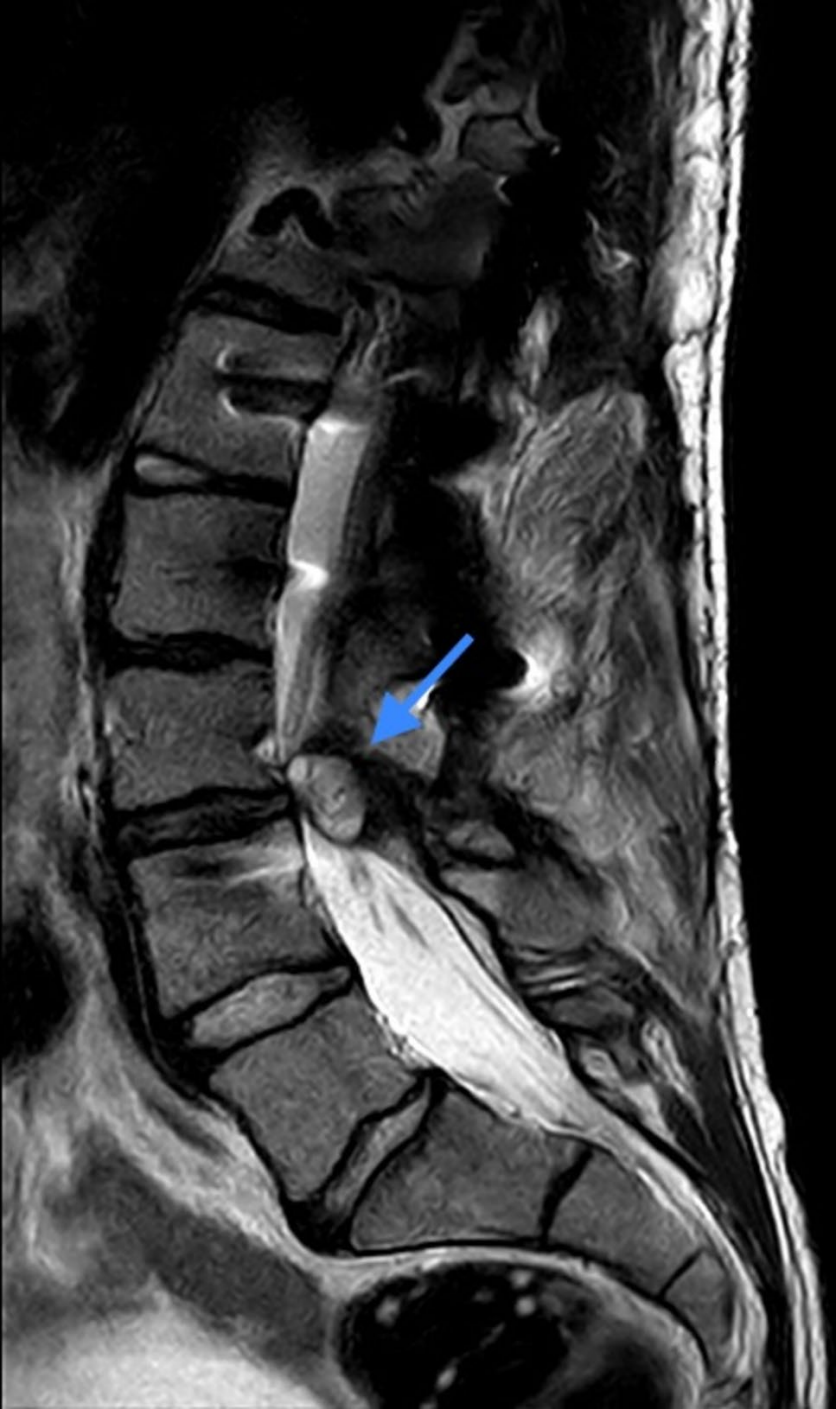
Sagittal magnetic resonance imaging showing lobulated epidural cystic formation (blue arrow)
